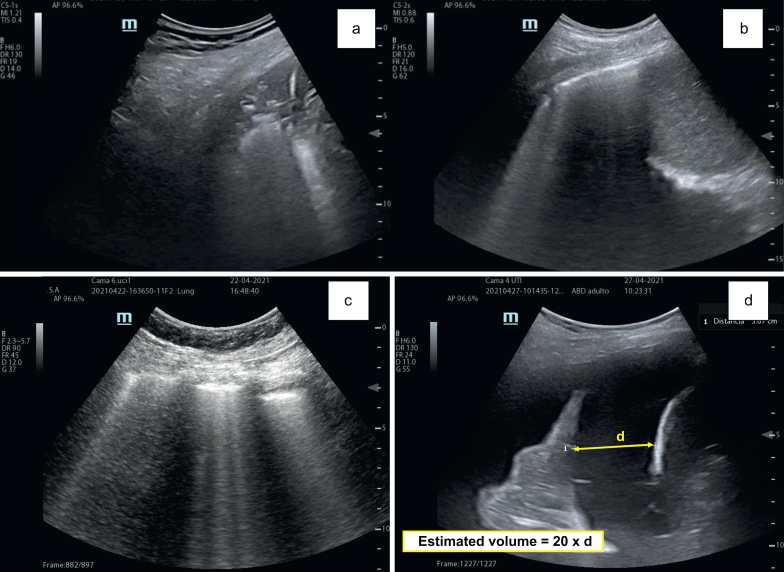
Consolidation and pleural effusion at lung ultrasound. The right lung base is seen with a convex probe. (a) Consolidation pattern with air bronchogram (indicated with arrow). (b) After 3 days, an improvement in lung ventilation is seen without consolidation pattern. (c) With convex probe in transverse plane at anterior level, B-lines of interstitial type secondary to weaning-induced pulmonary oedema (WIPO) are displayed. (d) With a convex probe in transverse position, perpendicular to the chest wall, at the level of the right base, extensive pleural effusion is seen. According to Balik's formula, a volume of 720 ml is estimated. Measuring the maximum separation between parietal and visceral pleural at maximal inspiration: 36 mm×20=720 ml.
